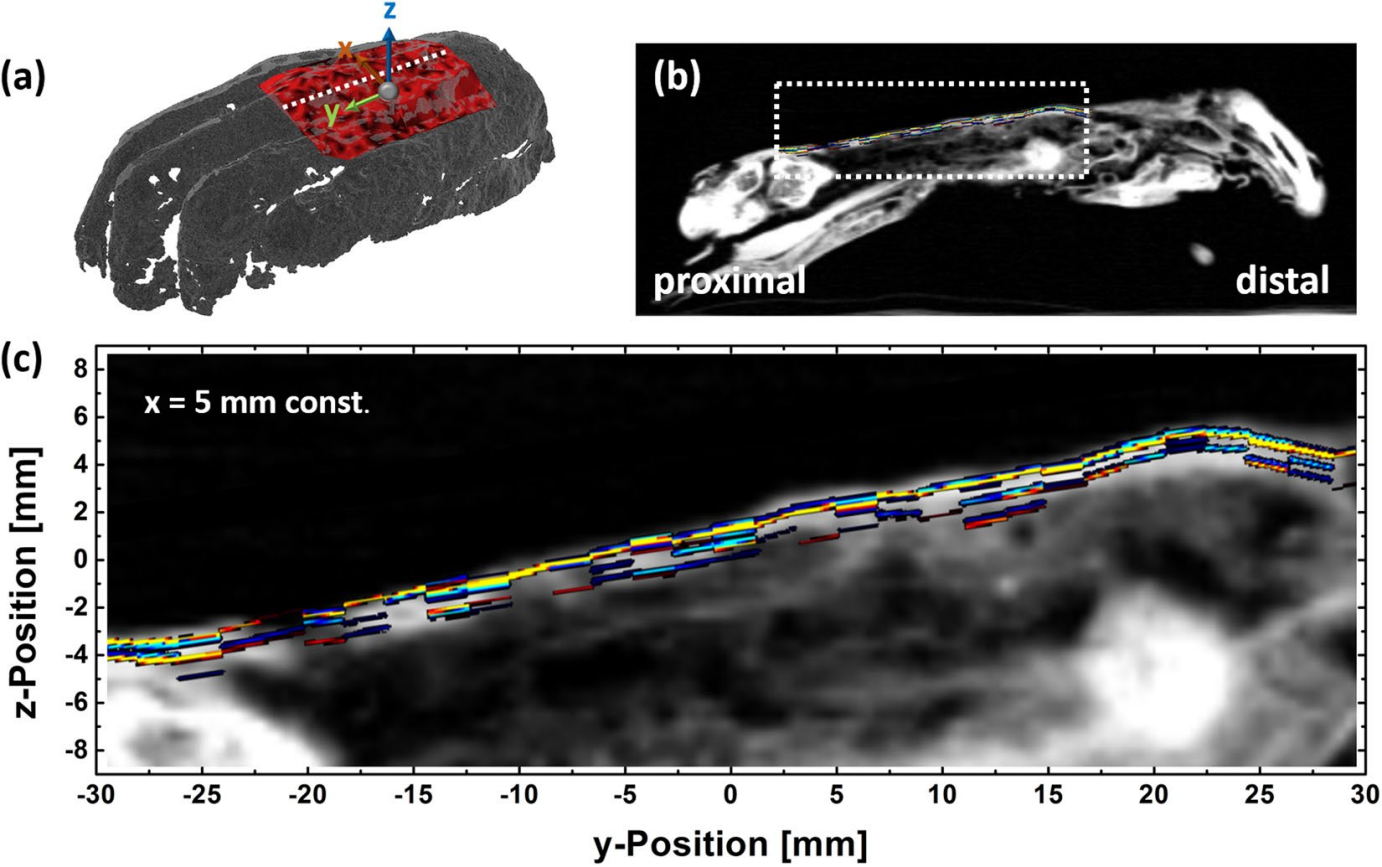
Comparison between reconstructed layers based on the THz measurement and a conventional CT scan. (a) The white dotted line in the 3D scan indicates the cross section at x = 5 mm, (b) THz measurement area in the CT scan. (c) Enlargement of the THz measurement area indicated by the white dotted rectangle in (b).
